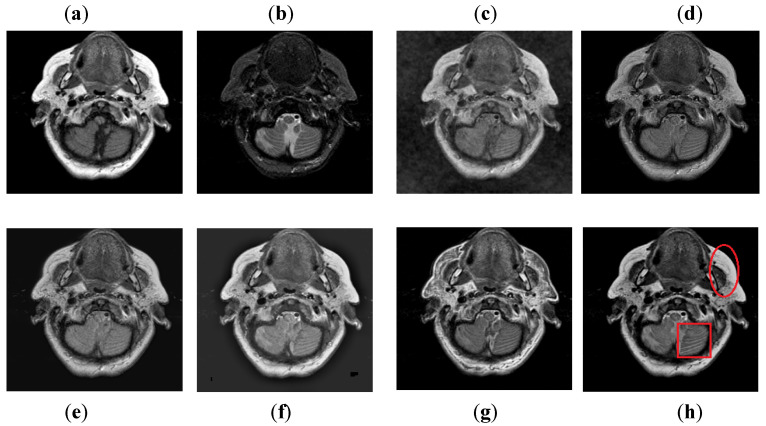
Data-3: (a) CT image, (b) MRI image, (c) DCT, (d) DWT, (e) NSST-SFT-PCNN, (f) CSR, (g) CNN, and (h) Proposed.
